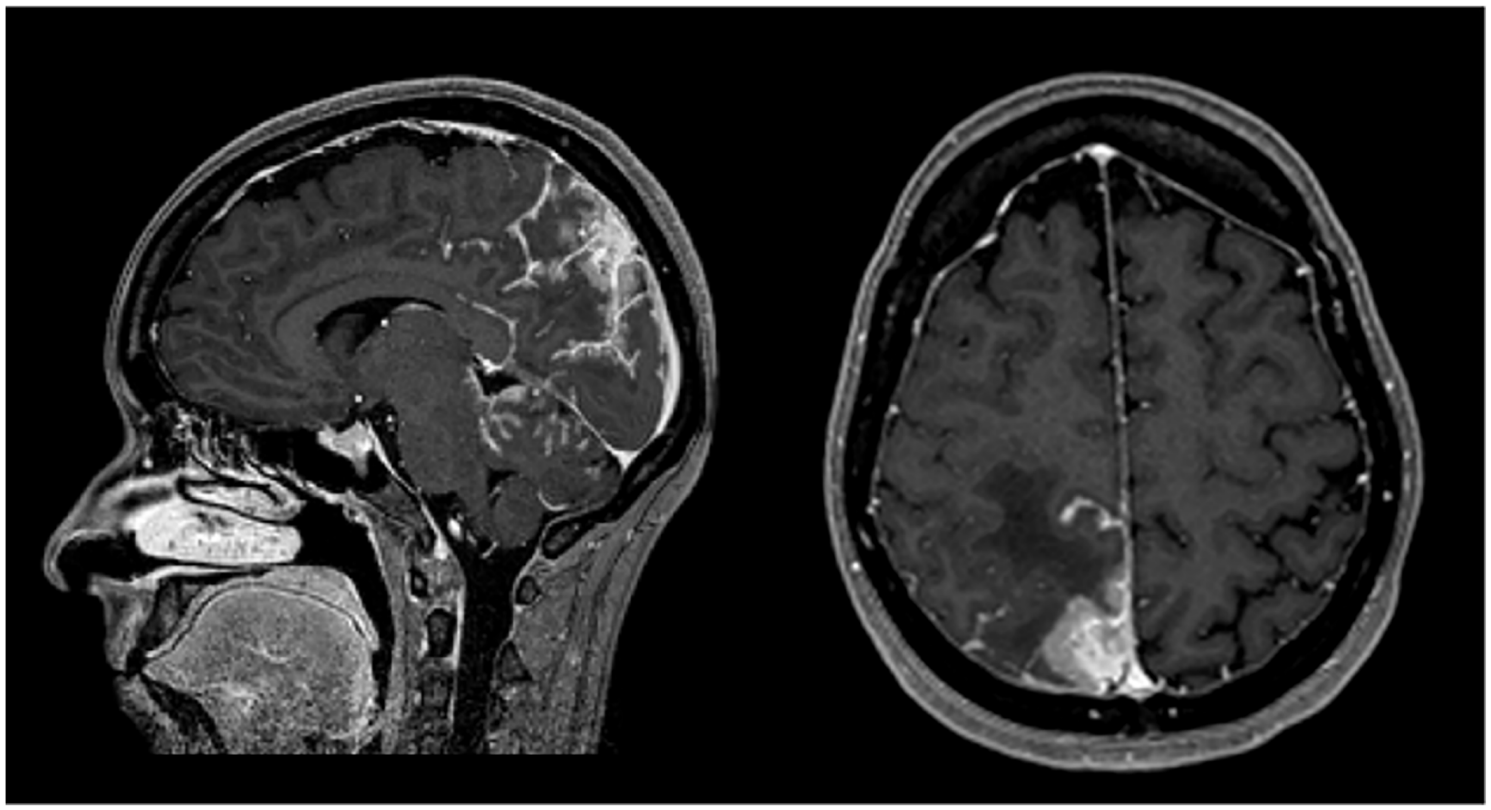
CE MRI at BM and LMC initial moment of diagnosis. Heterogeneous space-occupying lesion (right posterior parietal-parasagittal cortico-subcortical area), with vasogenic edema and sulcal effacement, compatible with a BM lesion, along with pachymeningeal carcinomatosis (right next to the first described BM).
